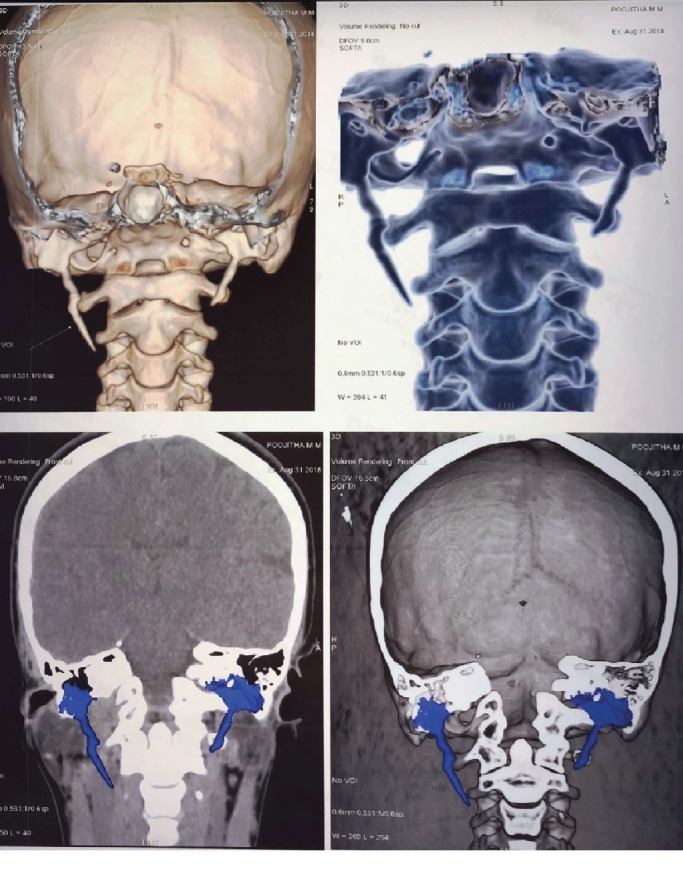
Computed tomography and 3D reconstruction.
